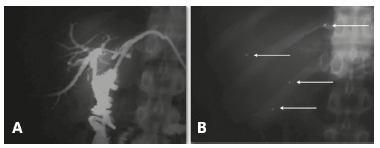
A) Stricture of the hepaticojejunostomy drained bilaterally; B) plain X-ray
